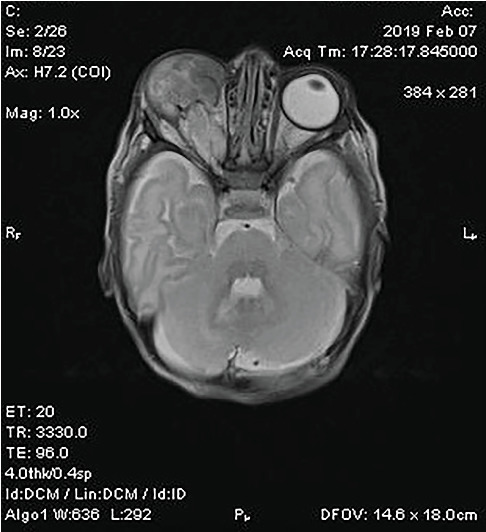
Axial T2-weighted MRI showing a large, heterogeneous, well-circumscribed mass in the right orbit, causing orbital wall remodeling without intracranial extension. The left orbit appears unremarkable.
